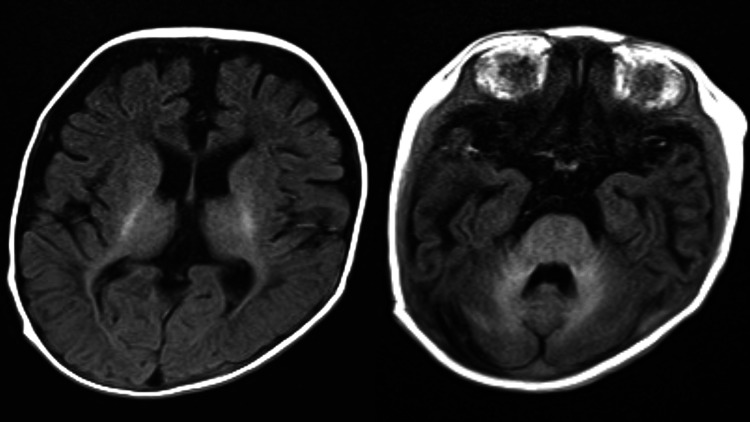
Case 1: T1-weighted images. Generalized brain atrophy is present with secondary enlargement of the ventricles and cisterns. However, there is no delayed myelination.
